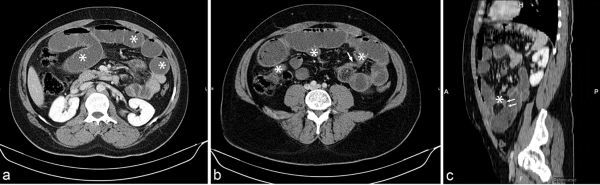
Contrast-enhanced axial CT image (a) demonstrates dilated small bowel loops (asterisks) with multiple air–fluid levels. Axial CT image obtained at a lower level and sagittal image (b, c) show the fibrous band at the transition point (arrows). The collapsed distal ileum (asterisks in b, c) is also seen.
